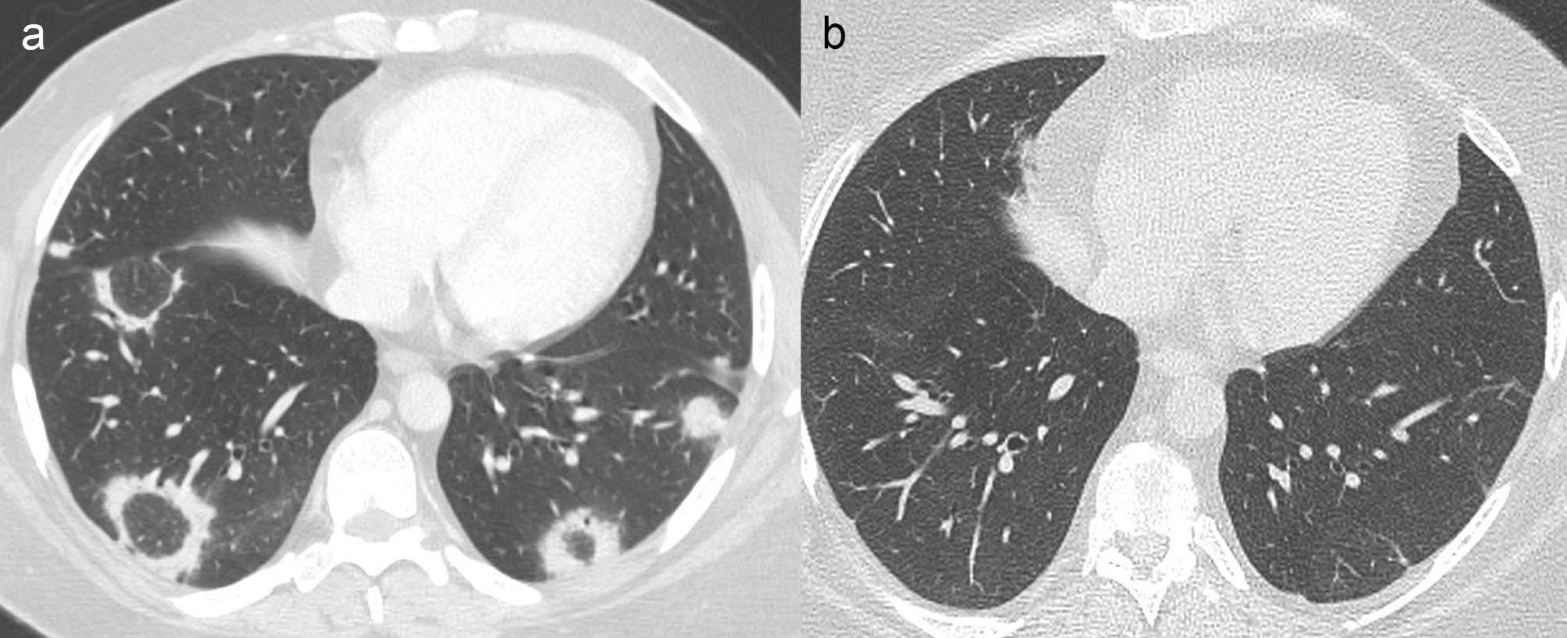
Drug-induced organizing pneumonia in an oncologic patient, with complete resolution after treatment. Drug-induced organizing pneumonia in a 29 y.o. woman with melanoma treated with nivolumab. Axial computed tomography (CT) image a shows bilateral perilobular consolidations with peripheral distribution in the lung bases. Axial CT image b performed after corticosteroid treatment shows almost complete resolution of the abnormalities, with some residual hazy ground glass opacities and subtle bands in the lower lobes
